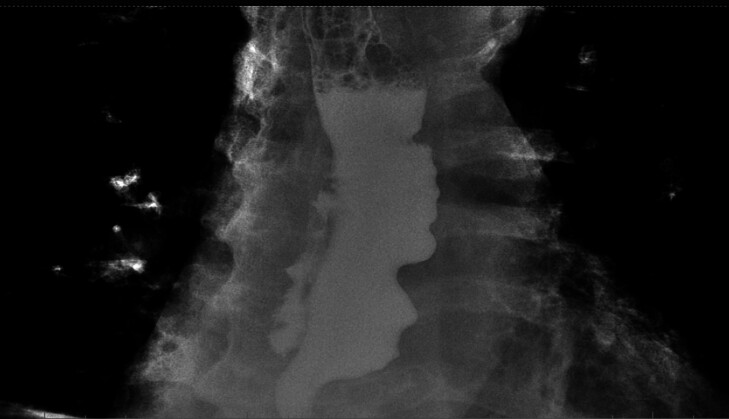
Esophageal X-ray with water-soluble contrast 24 hours after peroral endoscopic myotomy: esophageal double-lumen appearance.
